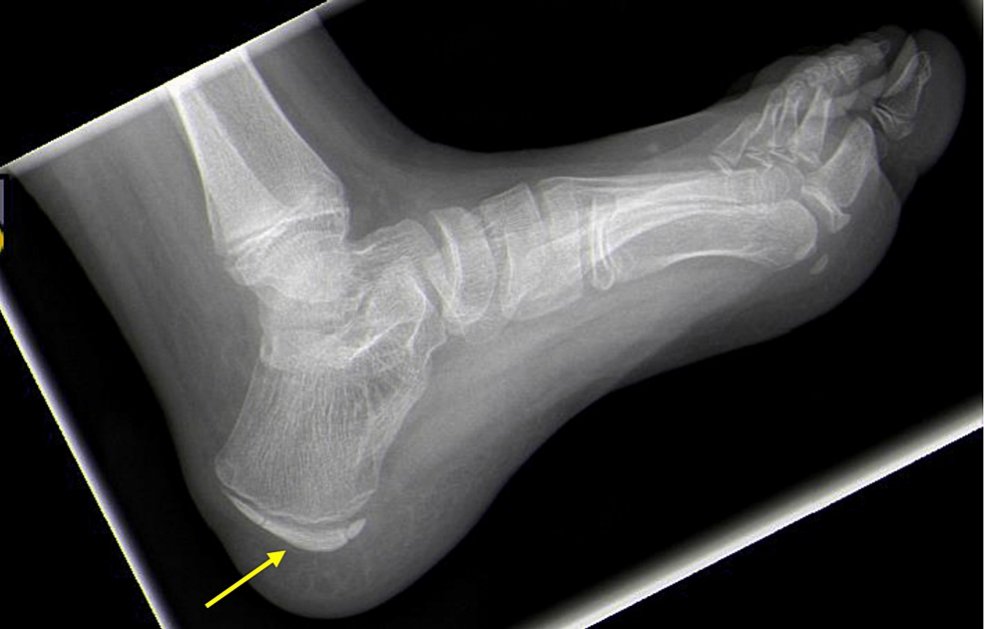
Lateral radiograph of the foot in a patient with Sever’s apophysitis. Note sclerosis and fragmentation of the calcaneal apophysis, which is not specific to Sever’s apophysitis and can be observed in asymptomatic patients.
